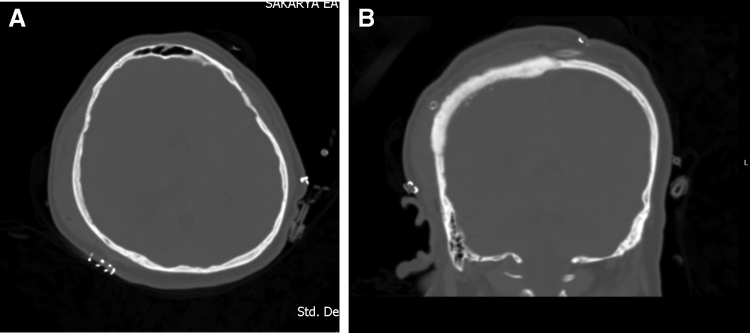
Immediate postoperative cranial computed tomography. (A) Axial bone-window image obtained on postoperative day 1. (B) Coronal bone-window image obtained on postoperative day 1.
